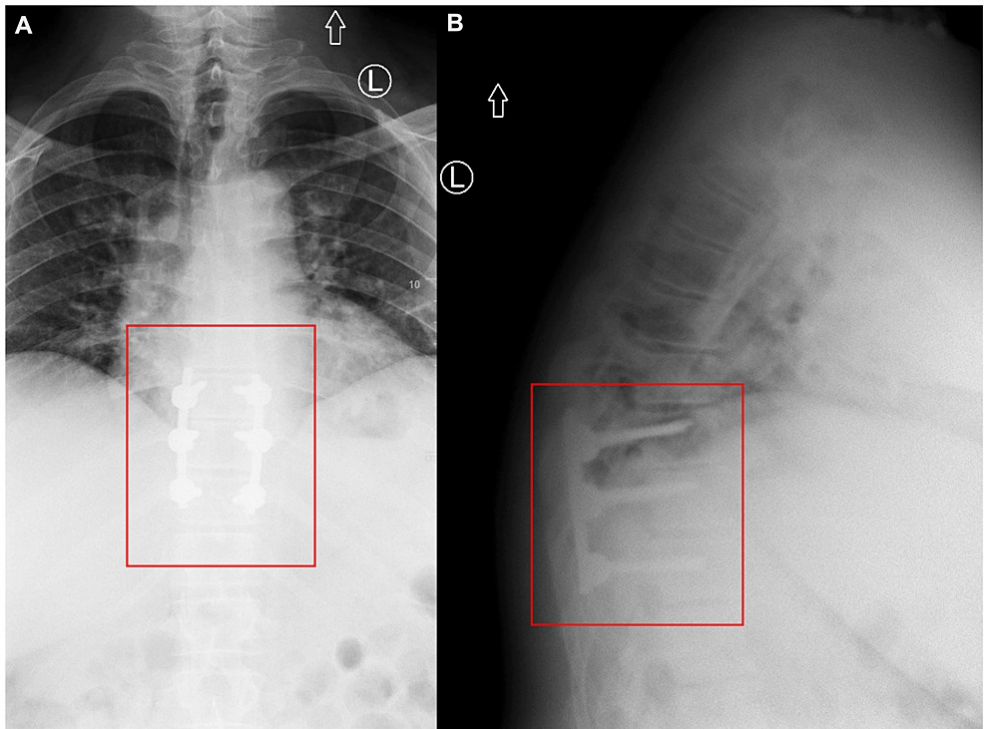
Postoperative thoracic X-rays. (A) Anterior-posterior X-ray showing pedicle screw placement from T9 to T11. (B) Lateral X-ray showing pedicle screw placement from T9 to T11. Red boxes indicate the area of interest.
